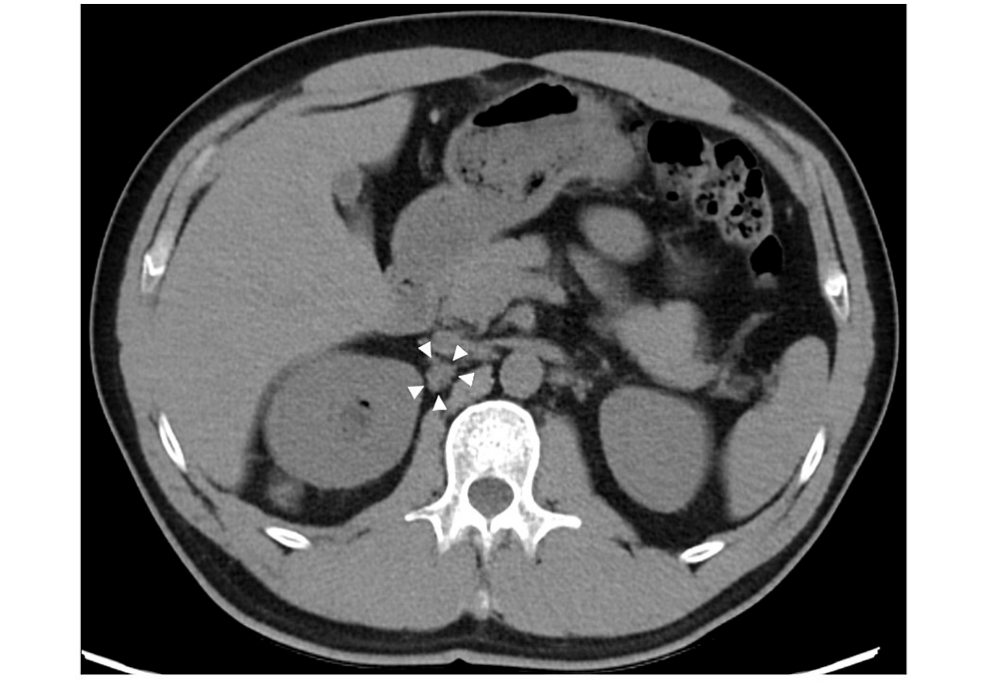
Possibly enlarged lymph node near the right renal hilum. A computed tomography scan performed three weeks after surgery displays a 5 mm, possibly enlarged lymph node near the right renal hilum (white arrowhead).
